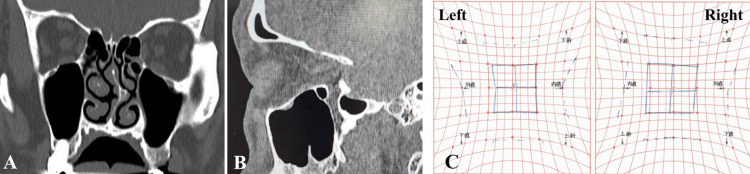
Postoperative CT and Hess charts. (A, B); Coronal and sagittal CT six months after surgery. The bone fragment observed before surgery disappeared and there was almost no bone defect in the left orbital floor. (C); Hess charts six months after surgery showing the good recovery of left eye movement.
